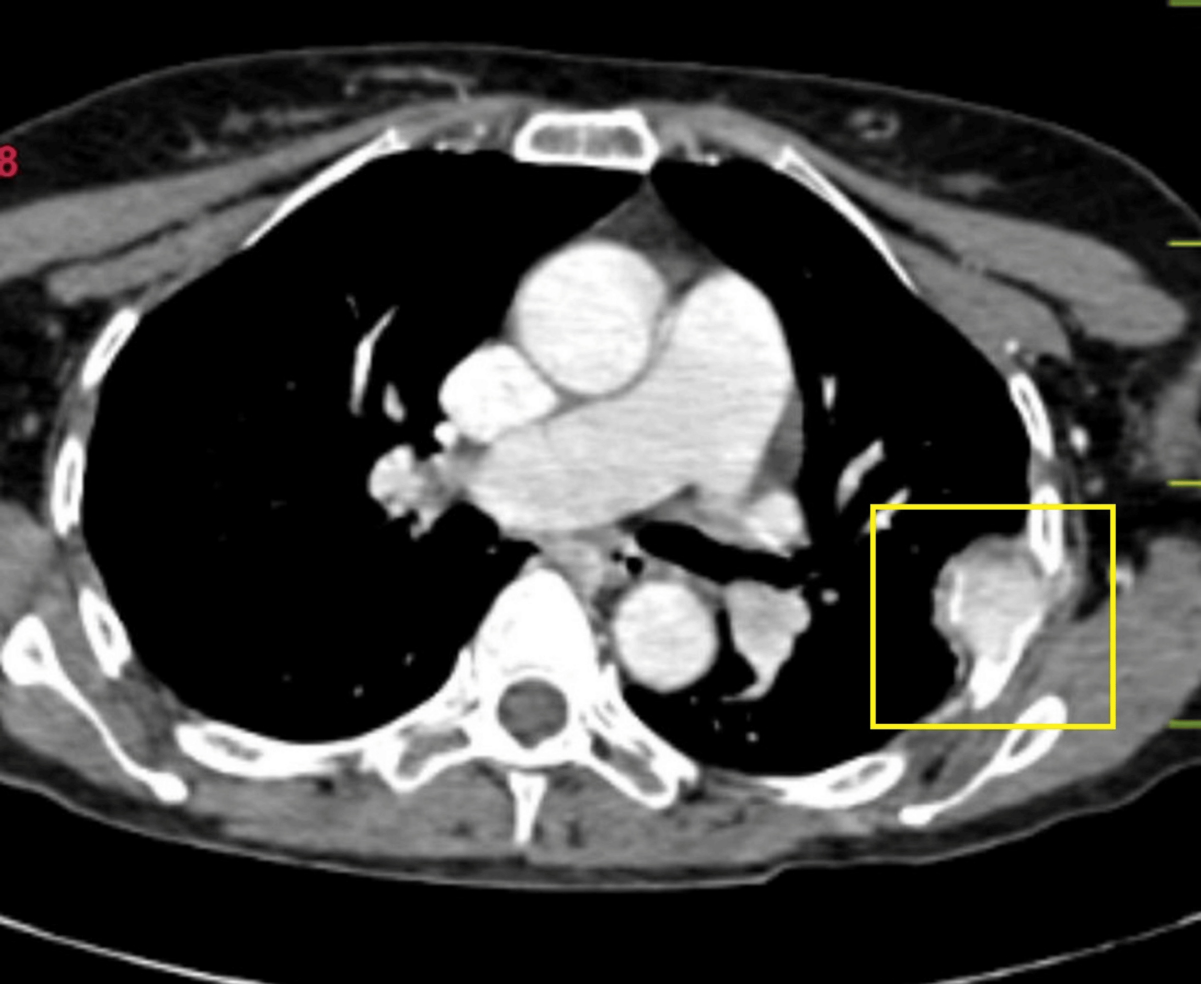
CECT showing the metastasis of the rib axial view showing enhanced soft tissue lesion arising from the fifth left rib with destruction of the involved rib CECT: contrast-enhanced computed tomography
